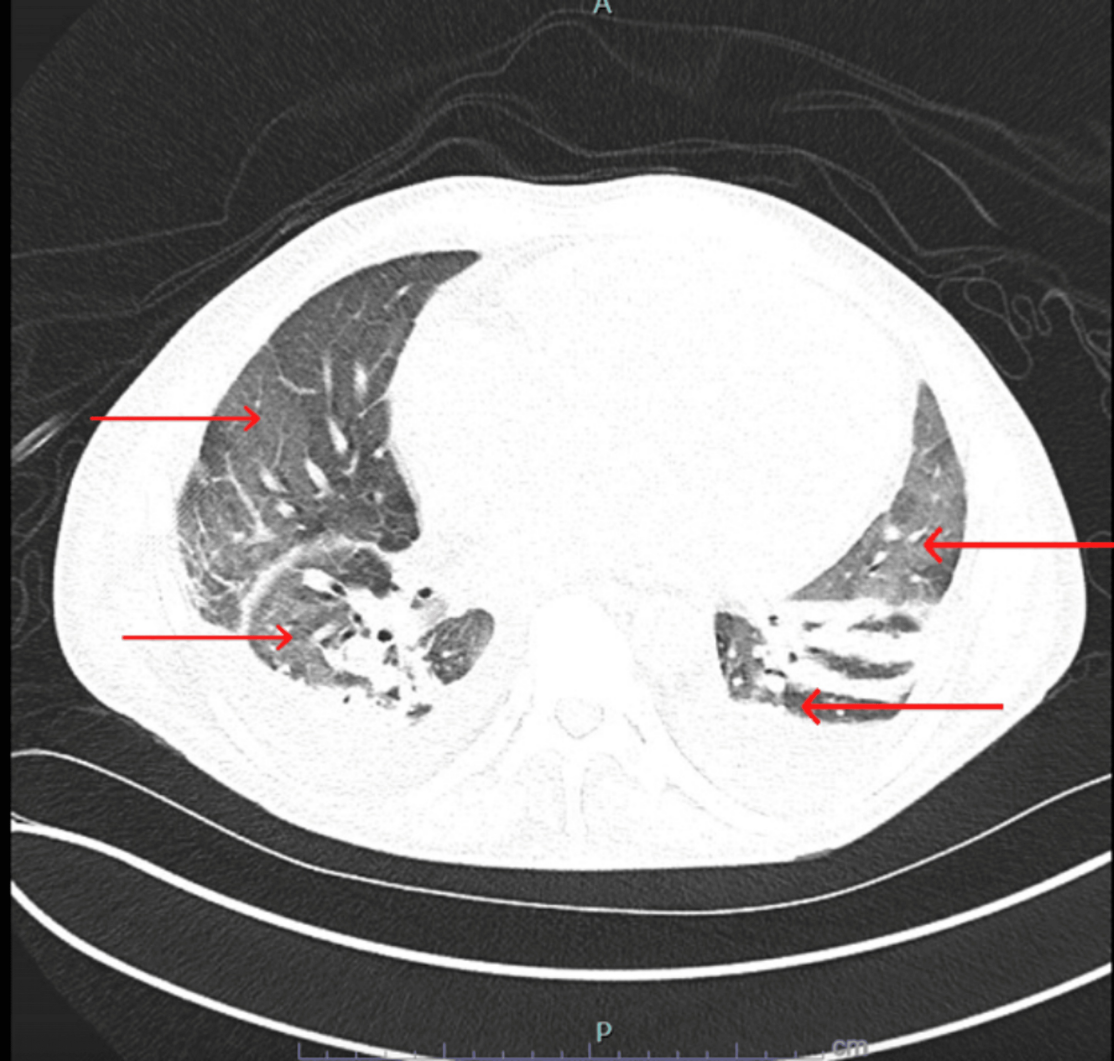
CT chest showing bilateral pleural effusions and patchy ground-glass infiltrates
